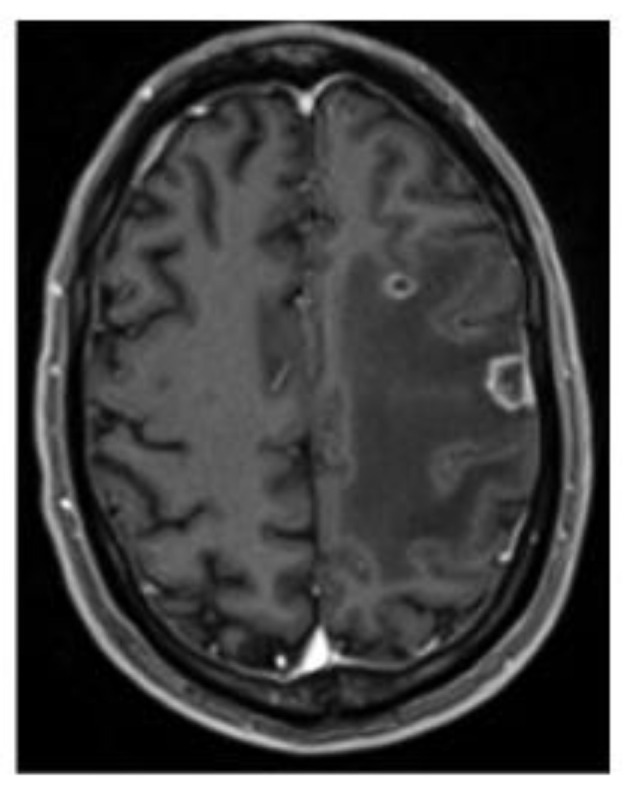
Brain axial T1 MRI with contrast, demonstrating two ring-enhancing lesions within the left-centrum semiovale with extensive adjacent edema.
